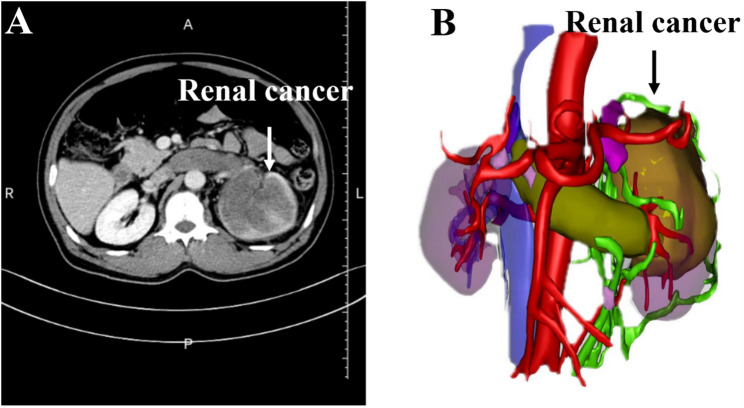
Three-dimensional image construction. A Patient’s specific two-dimensional CT image. B Patient’s specific three-dimensional image
